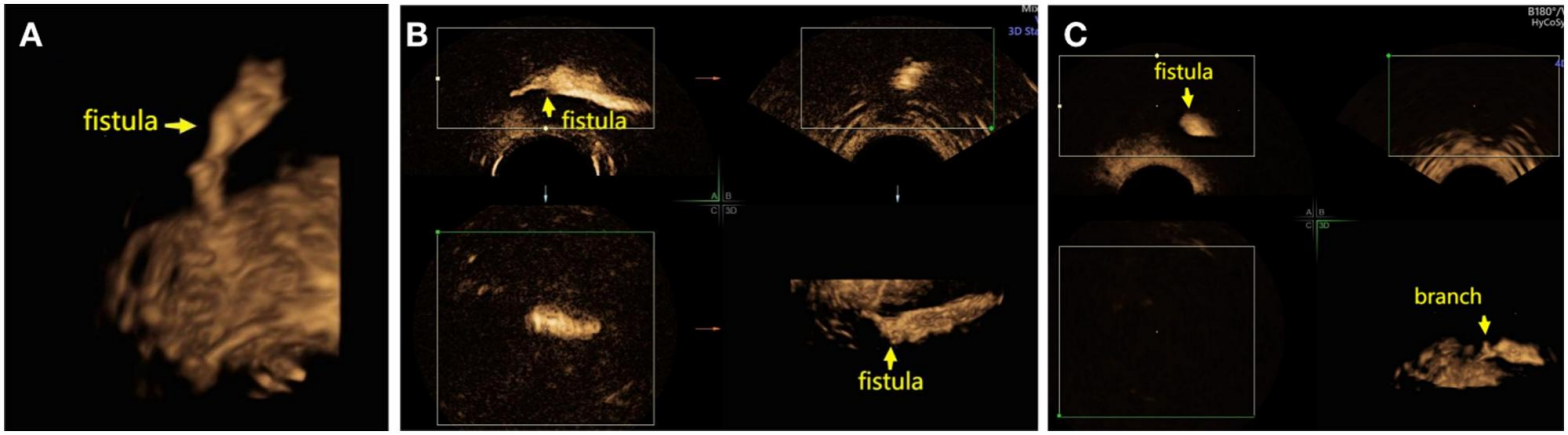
The tomographic ultrasound imaging technique is found to be helpful in tracing the pathway of fistulae. (A) Fistula imaging under SonoVue 3D transperineal ultrasound. (B) Sagittal, coronal, and transverse images of the fistula. (C) Imaging of branch fistula.
